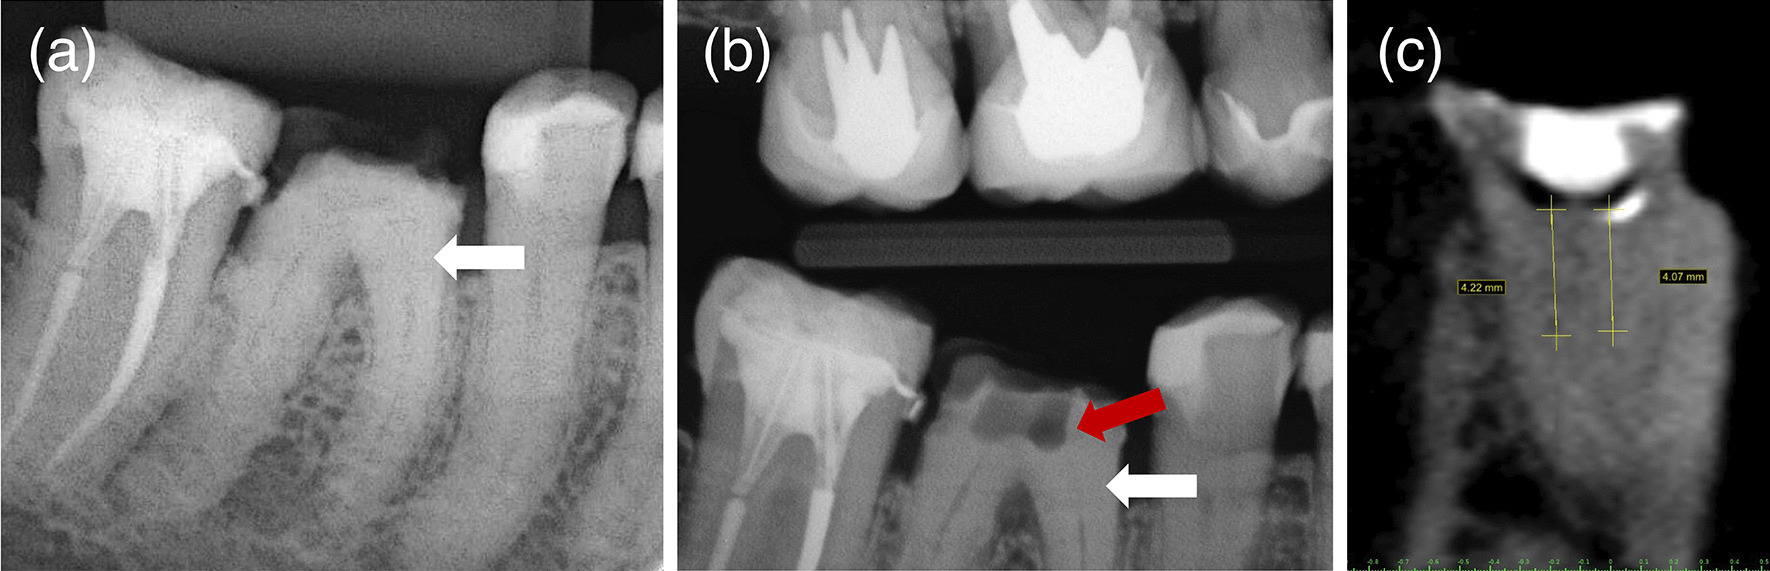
Diagnostic periapical XR image was suggestive of obliteration of the pulp canals (white arrow) (a). Bitewing XR image showing deviation in the mesial root (red arrow) towards the furcation area and the extension of the root canal obliteration (white arrow) (b). Cone Beam Computer Tomography (CBCT) image with estimated measurements of the obliteration’s length from the pulp chamber floor assuming the length of the 1.3 mm drill as the measurement parameter (c)
